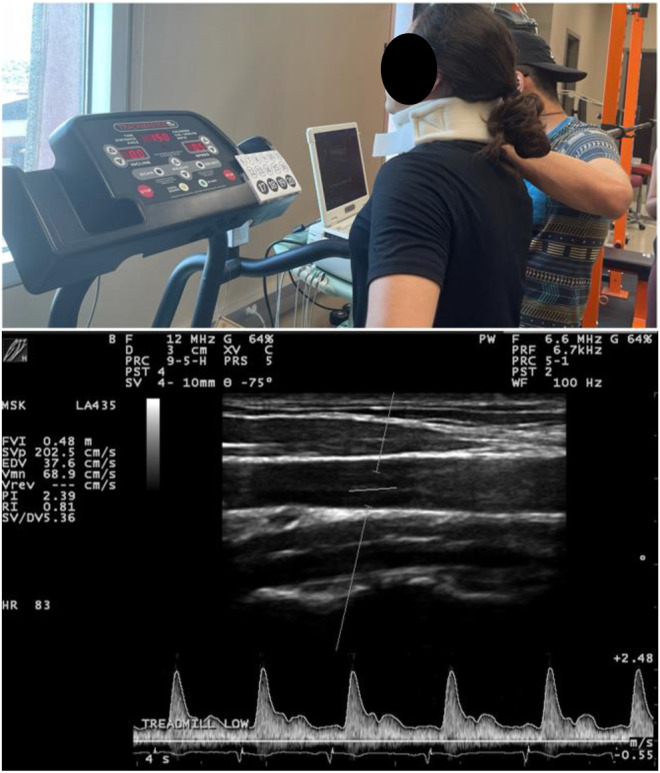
Typical ultrasound testing setup with neck probe holder utilized during all exercise testing and representative ultrasound image.
